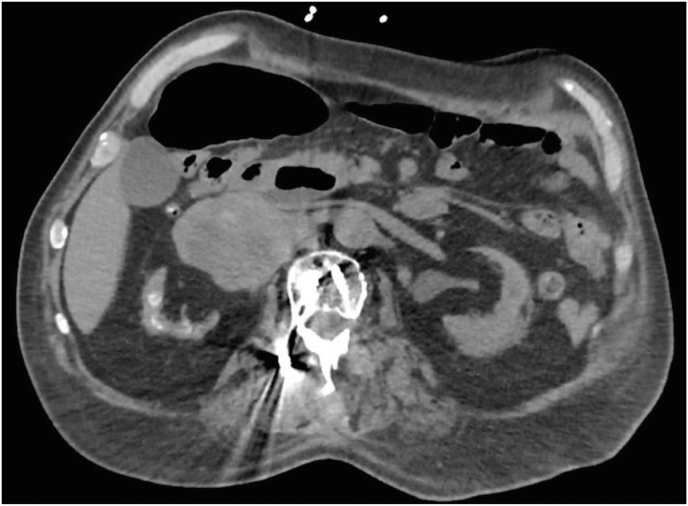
CT Abd/Pelvis demonstrating an 8.4 cm right renal artery pseudoaneurysm and renal atrophy.
